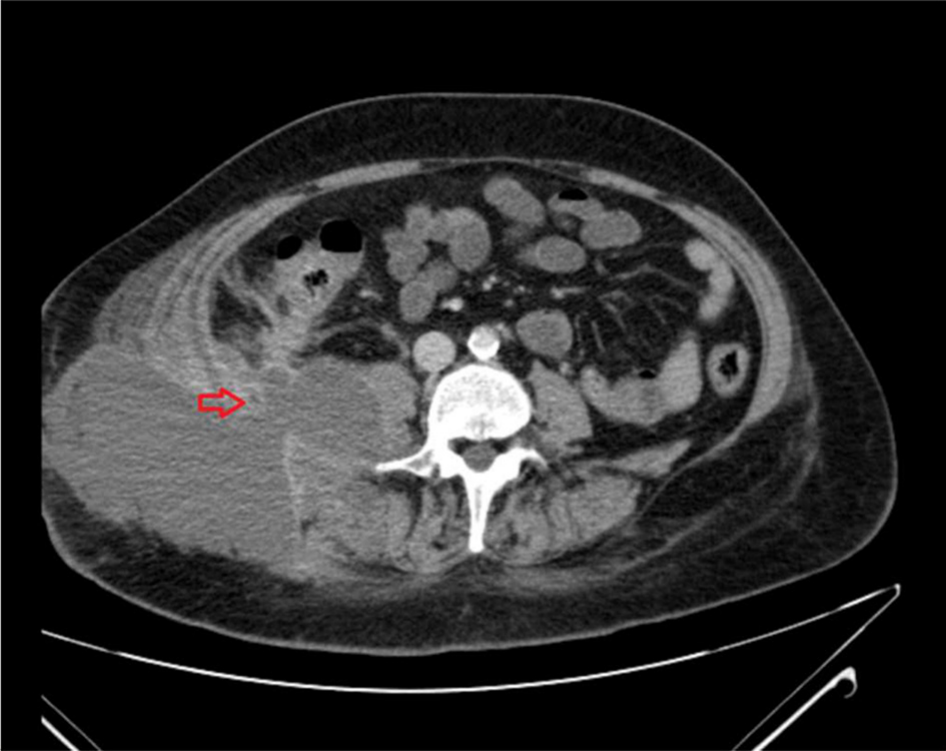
CT abdomen and pelvis (axial view) shows intra-abdominal abscess extending into the right flank/subcutaneous plane (red arrow).
